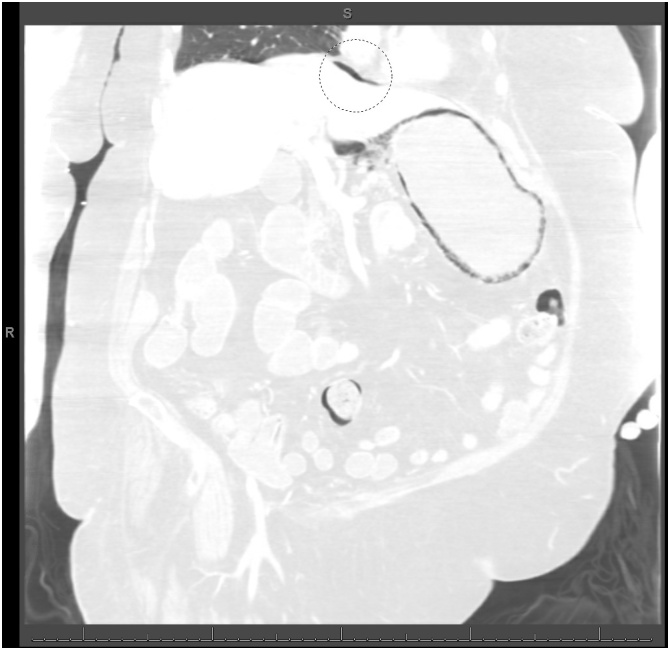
Computed tomography scan of the abdomen (lung window) showing extensive gastric pneumatosis and free intraperitoneal air superior to the liver (circle).
